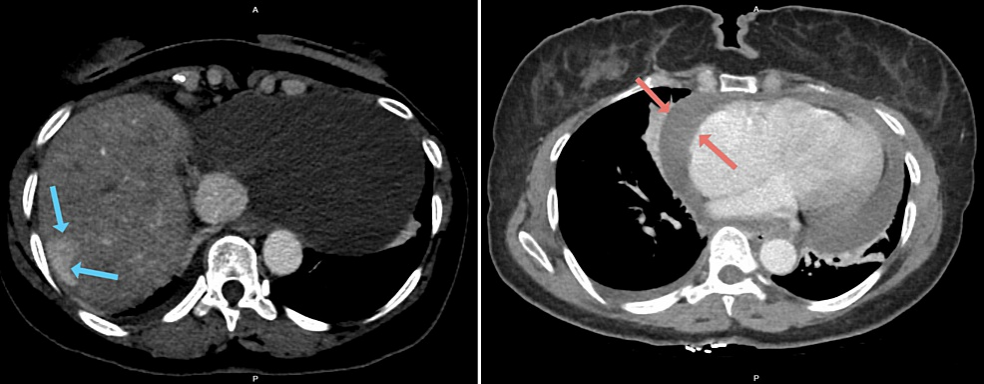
CT abdomen showing heterogeneous liver with multiple hyperenhancing foci (blue arrows) and large pericardial effusion (red arrows).
